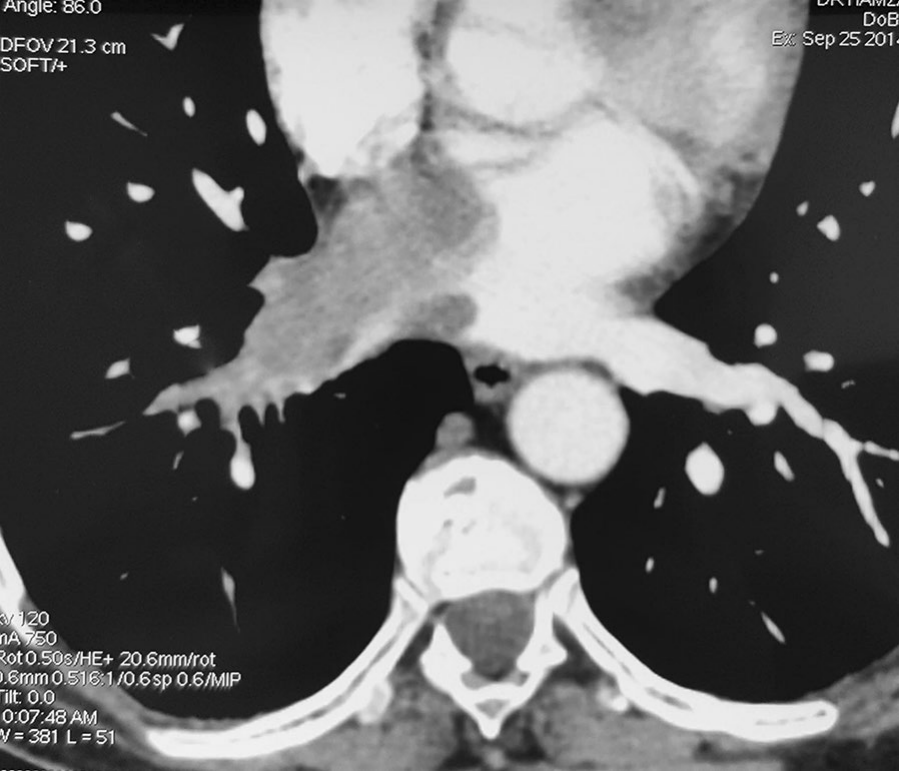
Computed tomography; Transverse section: 6 cm defect involving the right atrium and the right inferior pulmonary vein, which appears markedly enlarged
